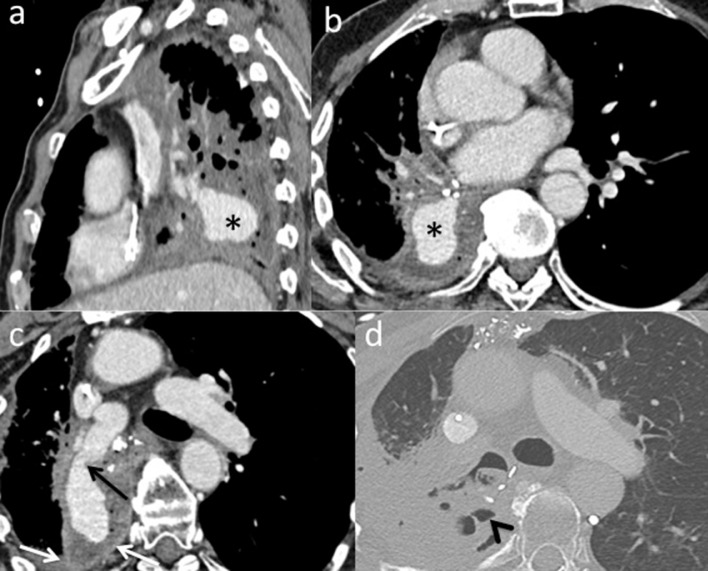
Chest computed tomography (CT) scans with arterial time injection in sagittal, axial section, in the long axe of the aneurysm sac (a-c), in mediastinal window and axial section in parenchymal window (d) show contrast media filling of the aneurysm sac in the right lower lobectomy cavity (*), feeding from the middle pulmonary artery (black arrow). Contrast-enhancement (white arrows) and air (arrow's head) within the lobectomy cavity suggest an infection.
